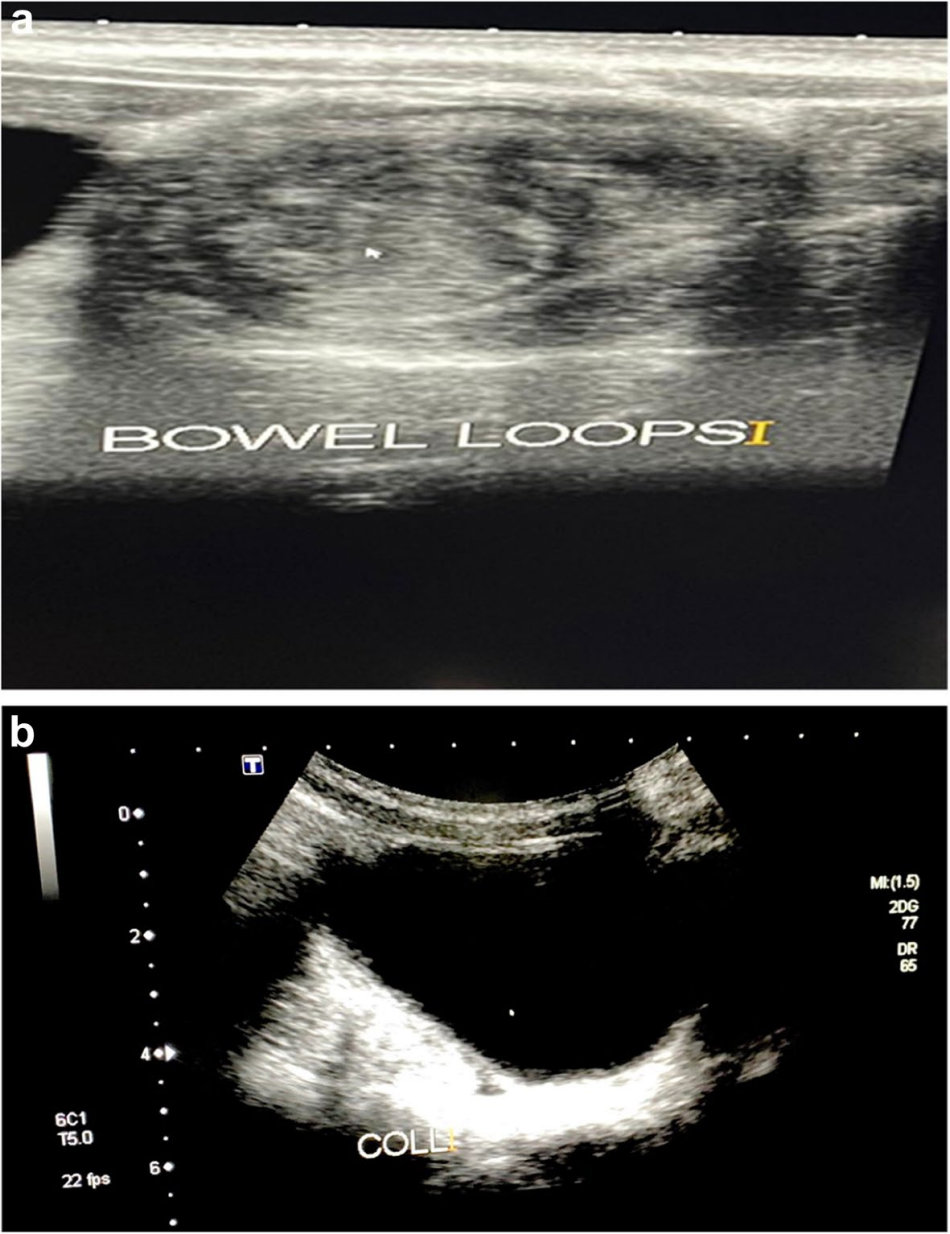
Transverse sonography of the patient’s abdomen performed during the emergency department visit (A) Ultrasonography showed a swirled pattern of alternating hyper-echogenicity and hypo-echogenicity in the right lumbar region. This represents the alternating layers of the mucosa, muscularis, and serosa seen in intussusception giving it a “target” appearance. B Moderate amount of free intraperitoneal fluid
